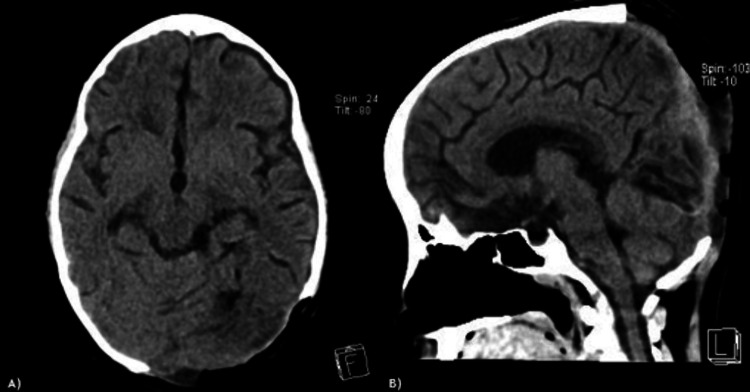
Brain CT images. A-B) Brain CT, 24 hours after surgery, shows complete resection without brain injury and expansion of the brain parenchyma. CT, computed tomography
